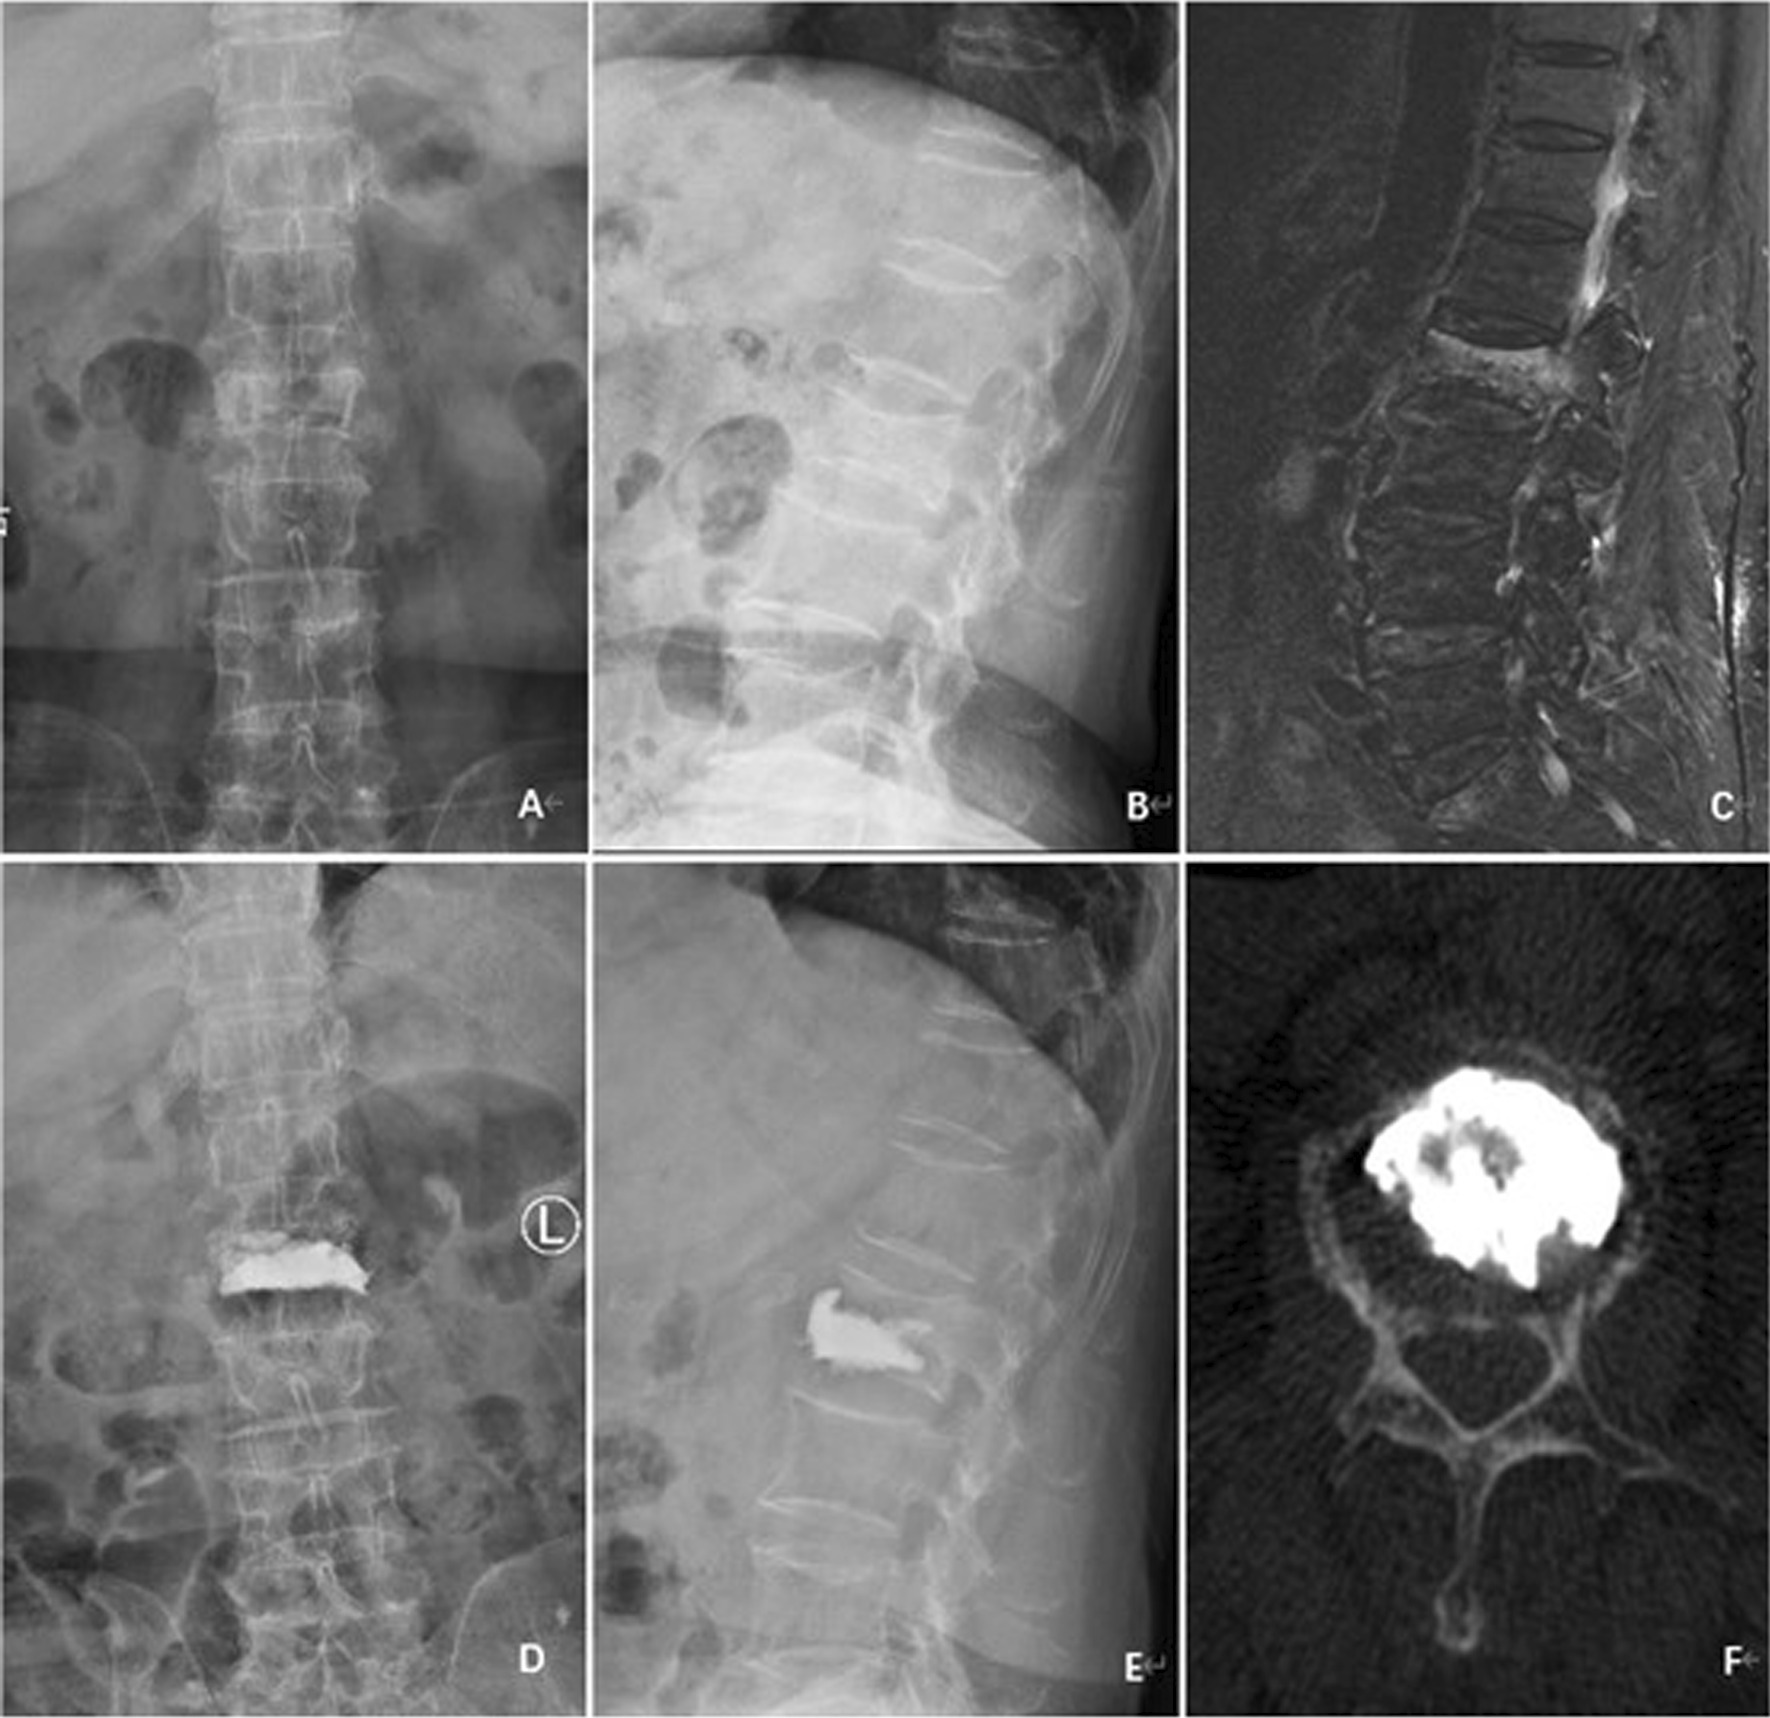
A 72-year-old female with single-level lumbar OVCF treated by unilateral PKP with directional bone cement delivery device. A, B Preoperative anteroposterior and lateral radiographs showed an L2 fracture. C, Sagittal T2W1 image showed the high signal within the vertebral body. D, E Postoperative anteroposterior and lateral radiographs showed an excellent bone cement distribution. F Postoperative CT scans showed that bone cement was mainly distributed symmetrically in the vertebral body
